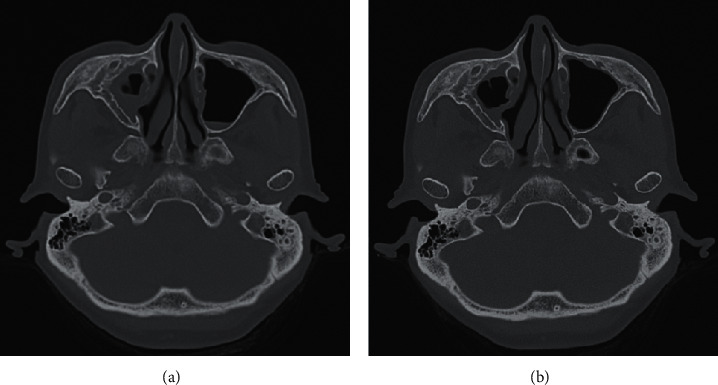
(a) Sinus CT before the administration of mepolizumab. CT shows fluid and mucosal thickening in the maxillary sinuses on both sides, predominantly on the right. (b) Sinus CT in 6 months after the administration of mepolizumab. CT shows a decrease in fluid and mucosal thickening of the maxillary sinuses on both sides.
